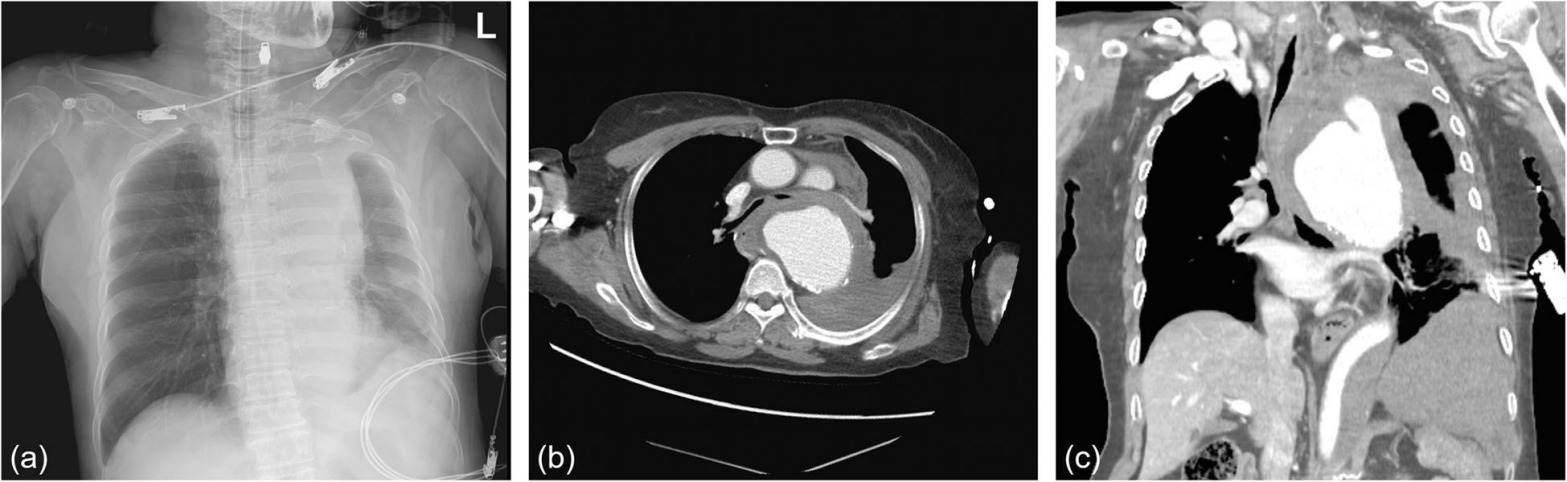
(a) Chest radiography and (b, c) computed tomography of the chest after the aneurysmal rupture. The maximal diameter of the aneurysm is 9 cm at the level of the carina
